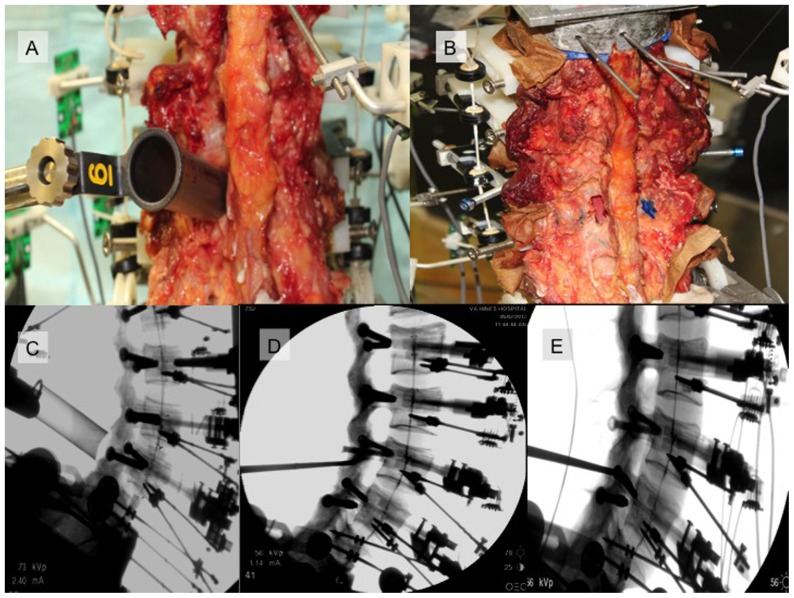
Images demonstrating testing of the minimally invasive surgical procedure. Unilateral docking of the METRx tube at L4/L5 is shown (A) as well as post-procedural anatomic changes following decompression (B). A radiograph demonstrating tubular docking is shown in C. Following complete removal of the ligamentum flavum and internal bony decompression, a curette was placed at the superior and inferior extent of decompression to confirm a “pedicle-to-pedicle” dural decompression.
